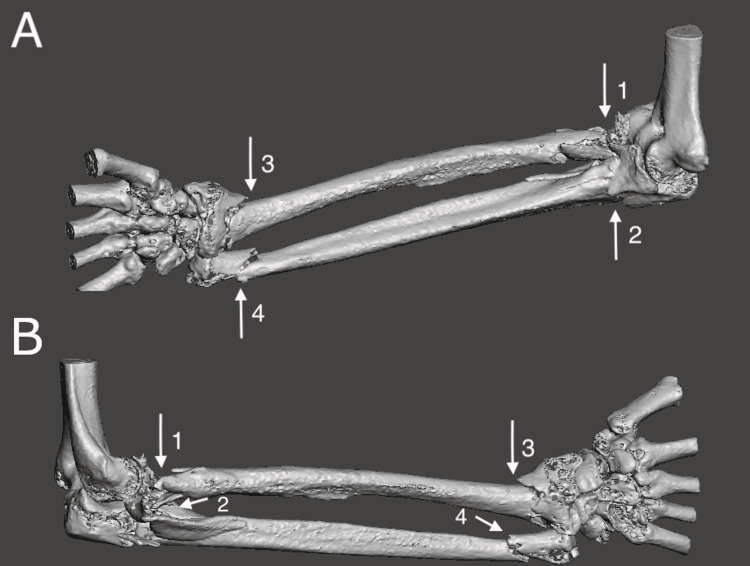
A: Medial view of the 3D CT reconstruction of the right forearm; B: Lateral view of the 3D CT reconstruction of the right forearm Arrow 1: Radial head fracture; Arrow 2: Olecranon fracture; Arrow 3: Distal radius fracture; Arrow 4: Distal ulna fracture
